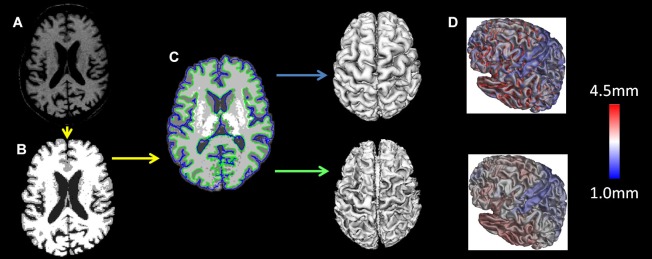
MRI preprocessing: the T1 images were (A) registered to the ICBM 152 template with a 12-parameter linear transformation, (B) RF inhomogeneity corrected and skull stripped, (C) tissue segmented and gray and white matter surfaces created and (D) tissue partial volumes estimated. Cortical thickness was measured as the distance between the white and gray surfaces in native space.
